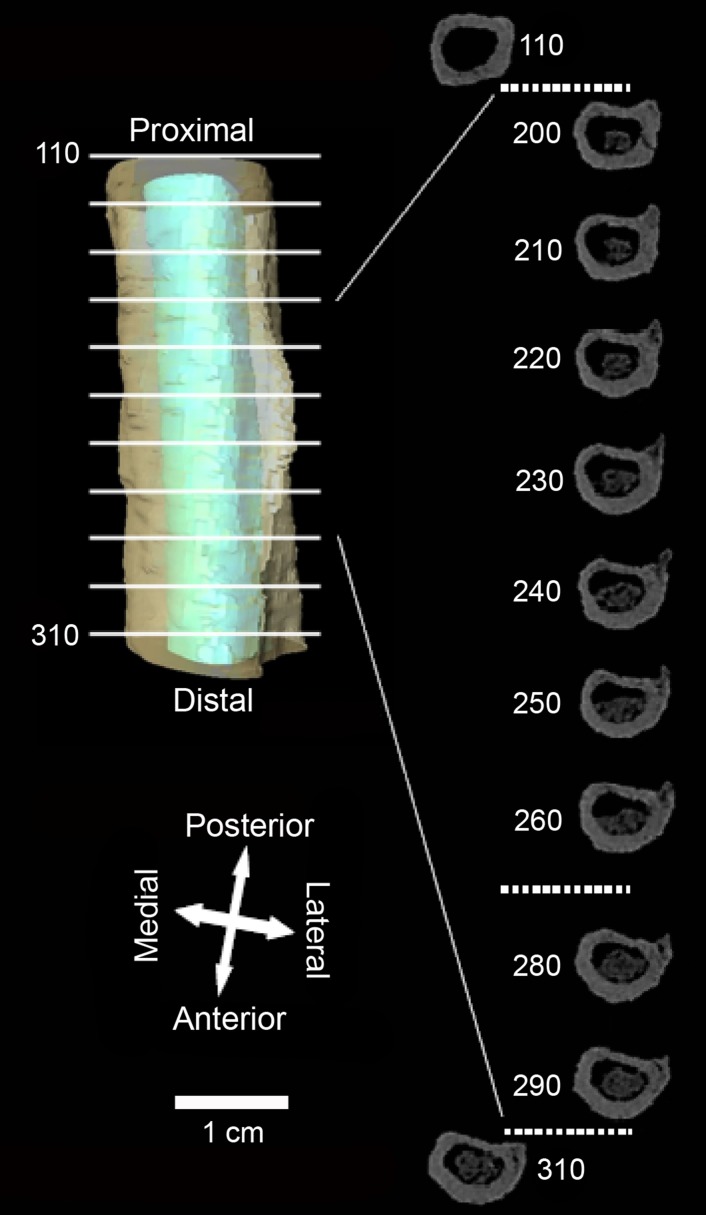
The enthesopathy modifying the posterior fasciculus of the deltoid muscle on the Tourville left humerus. Left: virtual reconstruction of the affected diaphyseal segment between horizontal cross-sections n° 110 and 310. In green: cortical bone, in blue: medullary cavity volume. Right: horizontal CT-scan of the same segment. Note both the substantial crest, which develops laterally and posteriorly, and the absence of any important relief at the area around insertion of the anterior muscle fasciculus.
